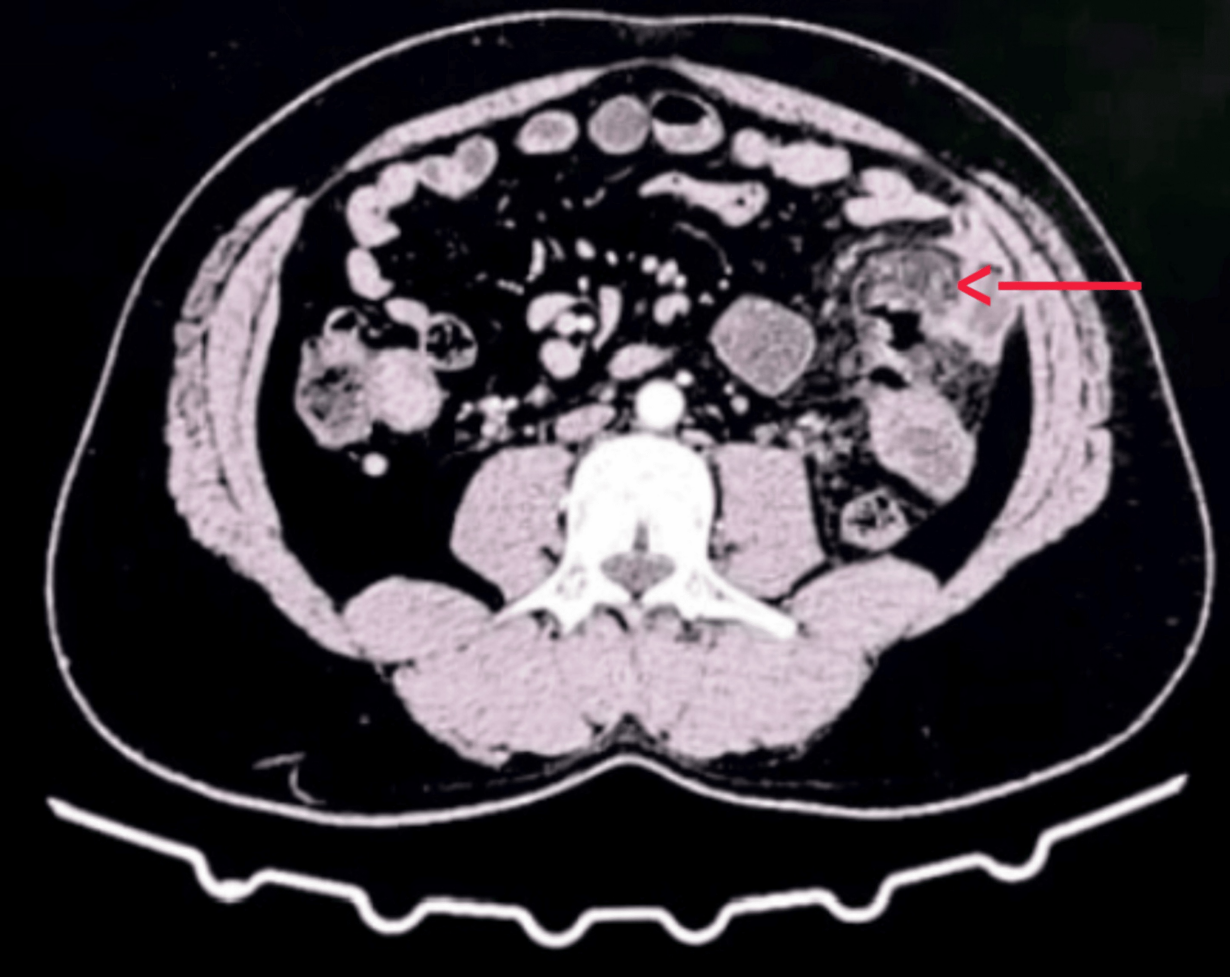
Contrast-enhanced CT abdomen showing increased mesenteric fat attenuation with mild soft-tissue stranding and a small adjacent fluid pocket near the jejunal loops (red arrow).
